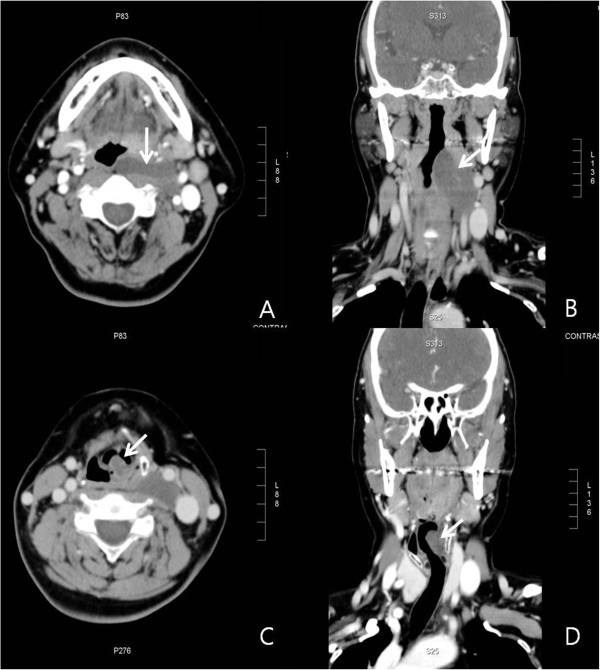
Computed tomography with contrast shows a well-defined homogenous mass lesion involving the parapharyngeal space (white arrows) (A, B) and left arytenoids (white arrows) (C, D).
